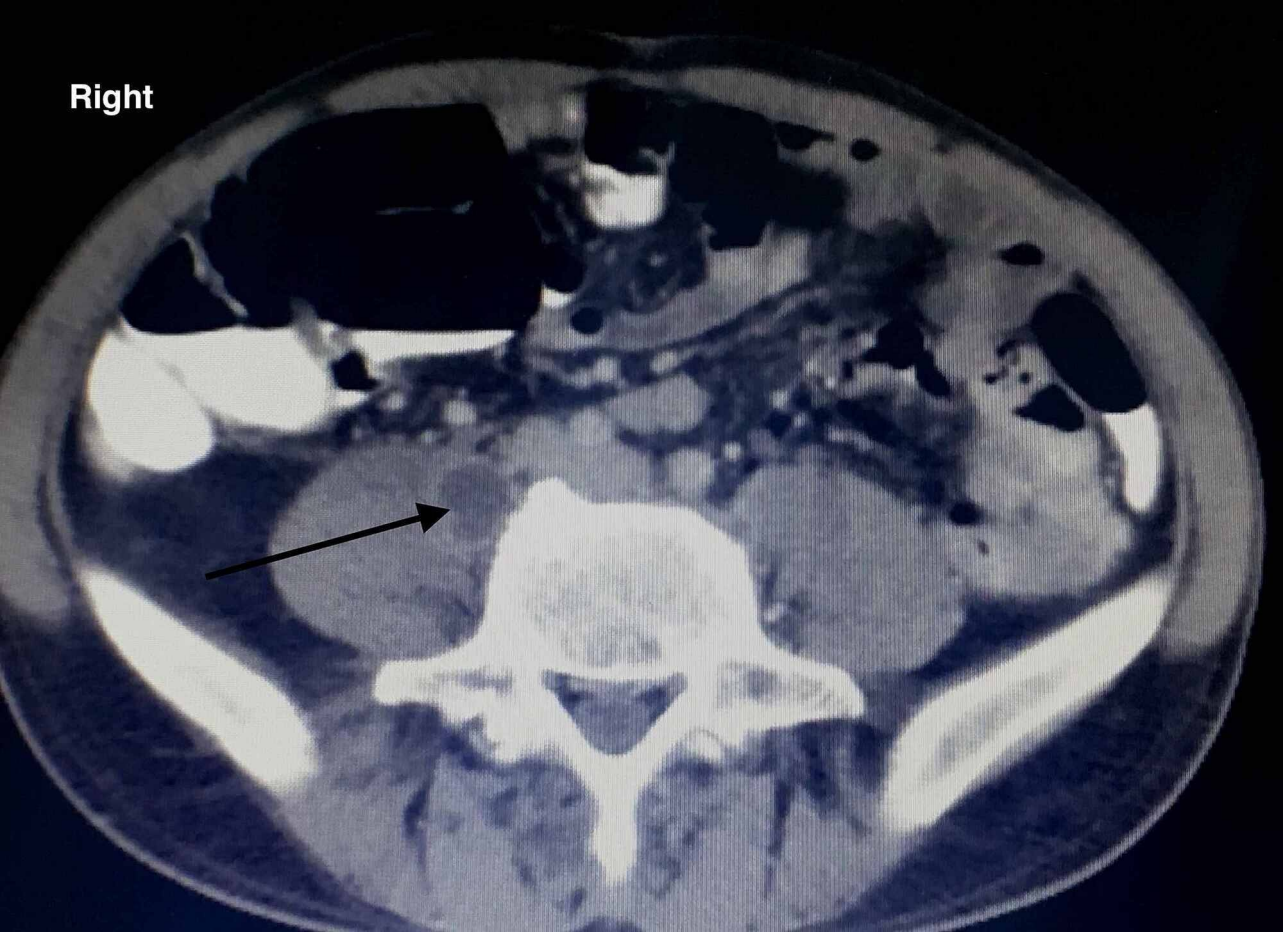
CT evaluation of the pelvis. An abscess of the right iliopsoas muscle is noticed, indicating the local spread of the infection.
